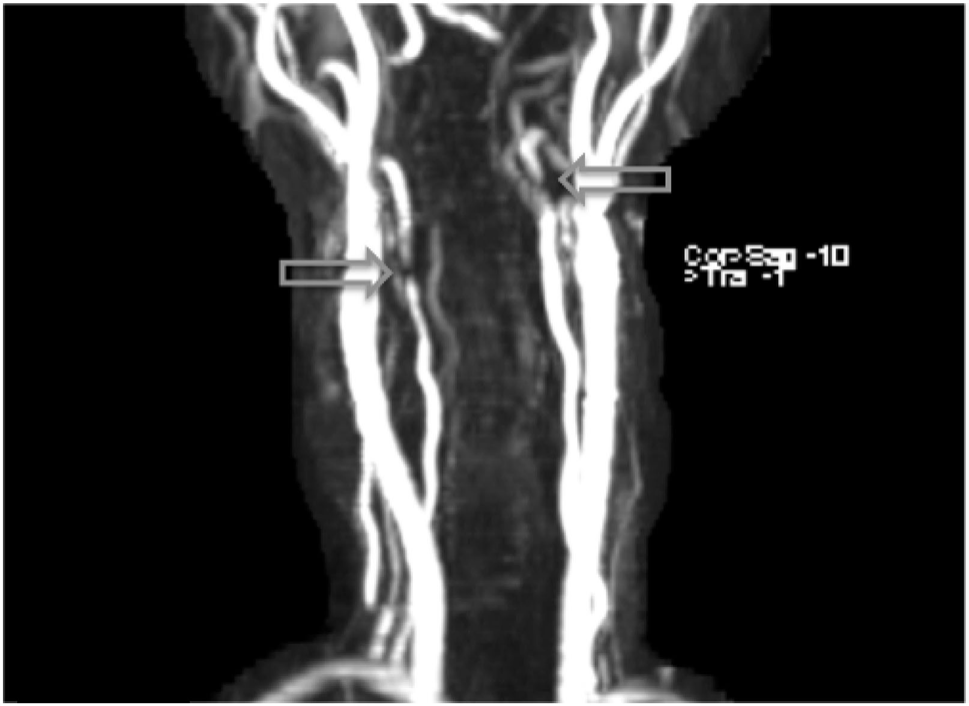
Arterial dissections noted in both vertebral arteries on MRI angiography following the patient’s second week on lenvatinib (14 mg) treatment.
